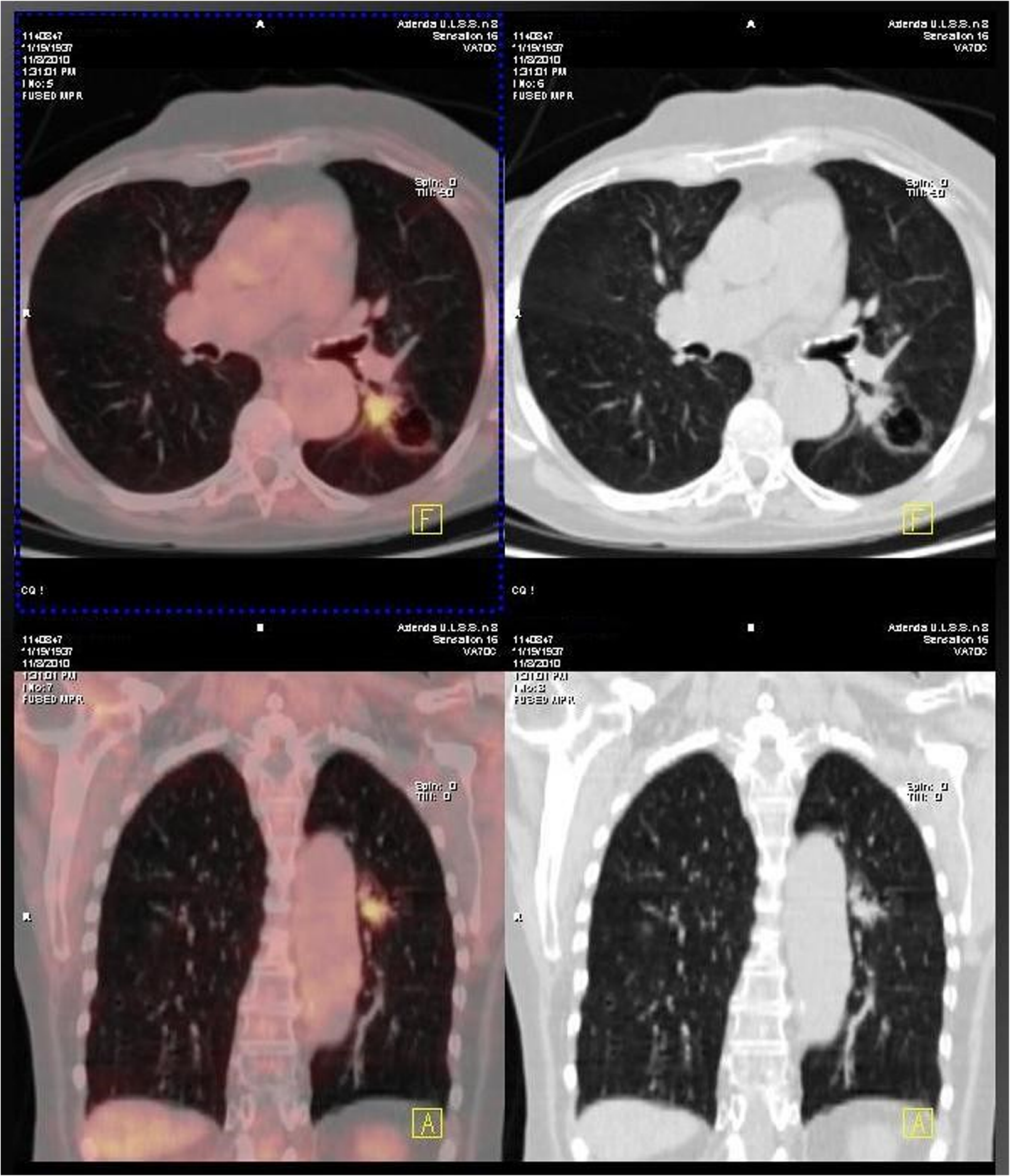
PET/CT incidental detection of left pulmonary adenocarcinoma 31 months after distal pancreatectomy for pancreatic cancer
